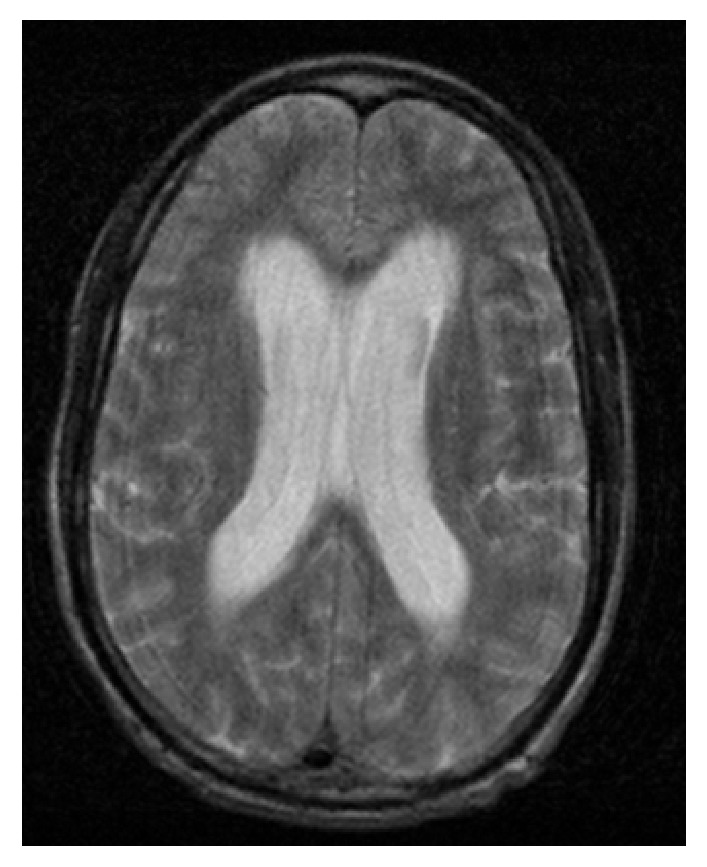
Head MRI showing moderate hydrocephalus and increased T2 flair between the gyri, concerning for meningitis.
